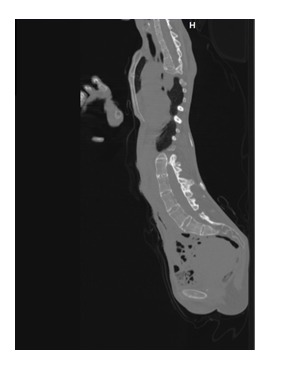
CT aspect. Marked kyphoscoliosis deformities. Lateral view.
